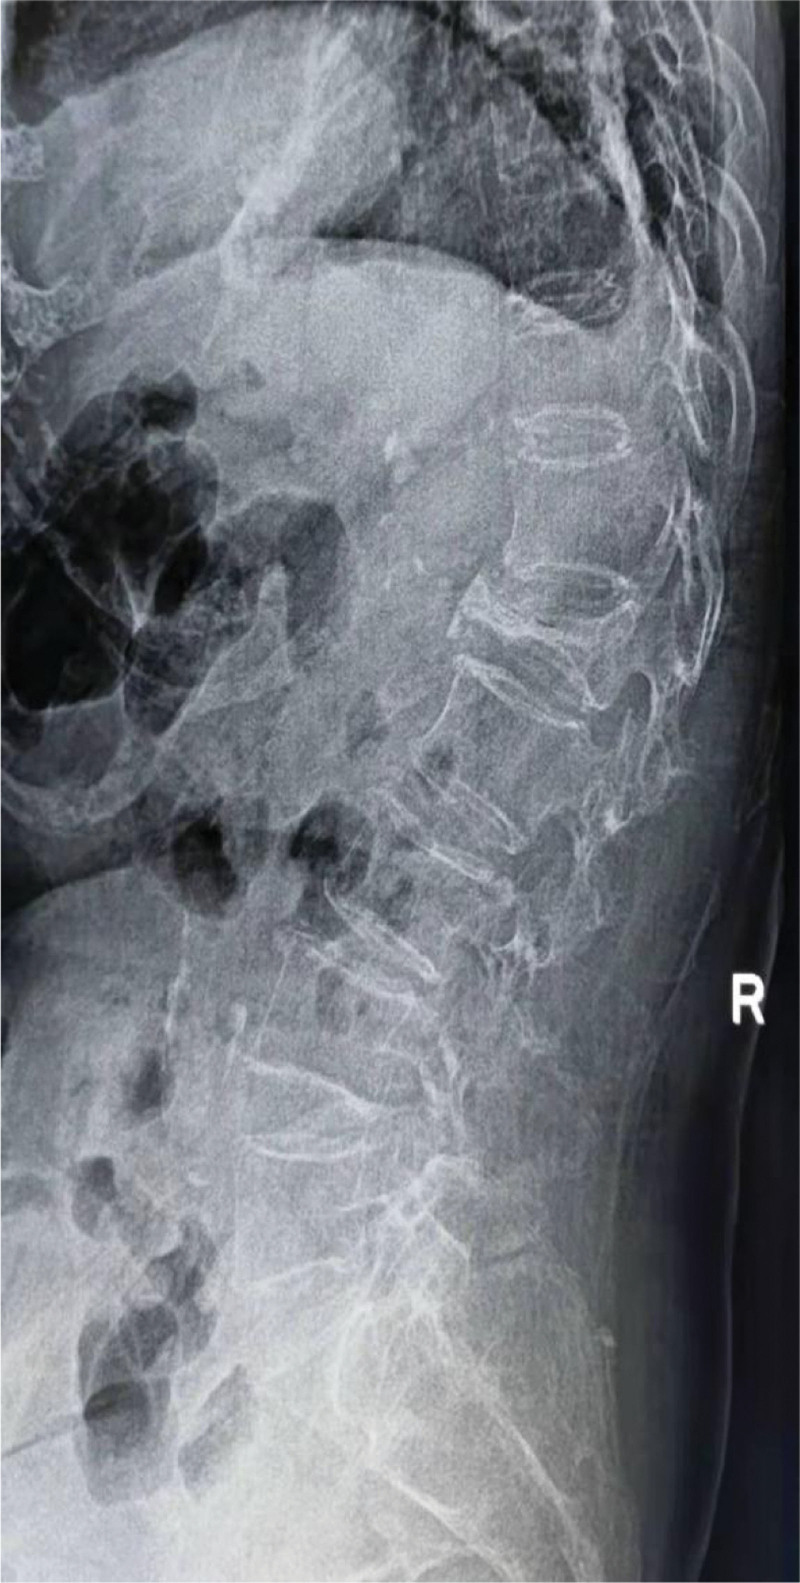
(A) An 85-year-old woman with osteoporosis presented with lumbar pain for 2 days. DR revealed compression fractures of the L1 and L3. (B) T1-weighted image indicated low signal intensity within L1 and L3. (C) T2-STIR image indicated high signal intensity within L1 and L3. (E) PKP was implemented in L1 and L3 in our medical institution, and L2, a sandwich vertebrae was also formed, as shown in (D) and (E). (G) One month after the initial treatment, the patient again sought medical attention due to low back pain. T1-weighted image manifested low signal intensity and T2-STIR image indicated high signal intensity in L2, a sandwich vertebral body, As shown in (F) and (G).
